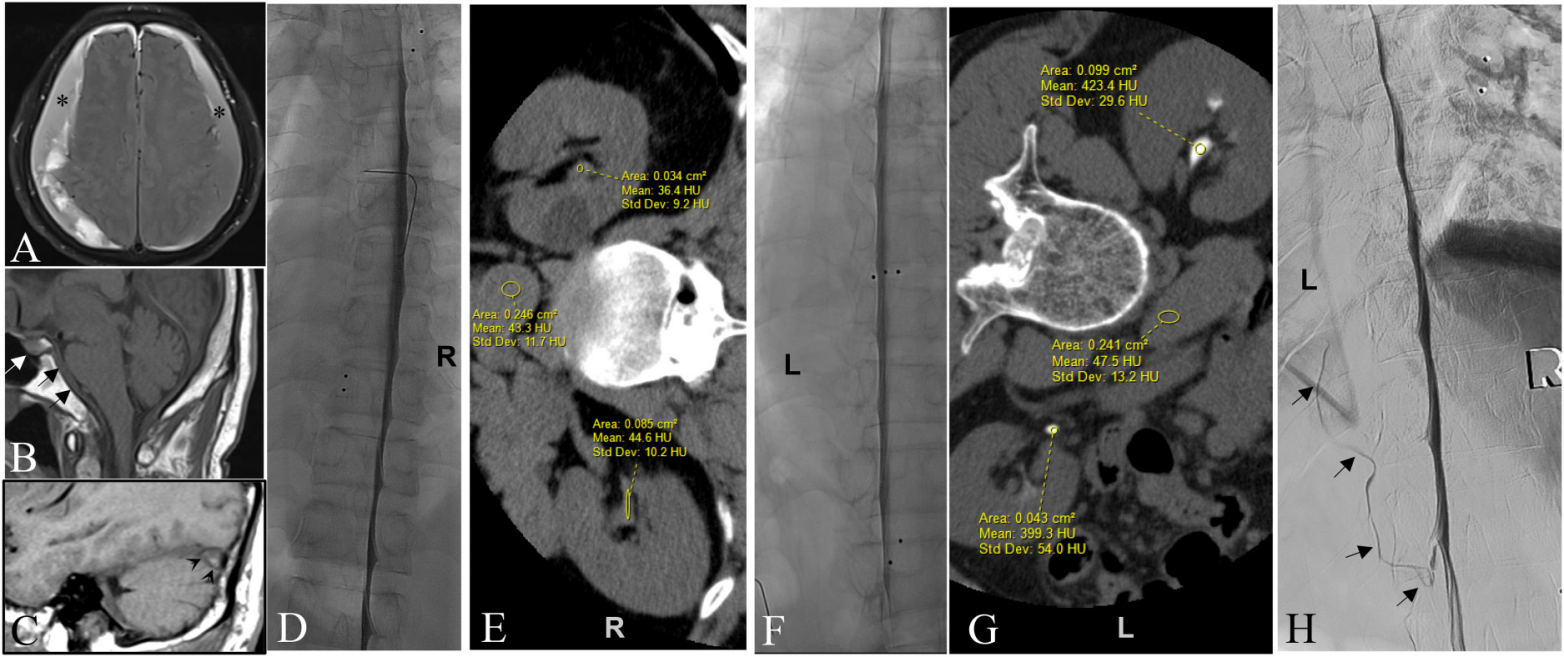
Patient 11. Showing differential rates of renal excretion associated with right versus left decubitus DSM–CT pair predicting sidedness of the culprit CVF . (A) Axial FLAIR image showing large bilateral subacute SDHs over the convexities of the brain (asterisks). (B and C) Midline and paramedian sagittal T1 images of the brain respectively showing enlargement of the pituitary (white arrow), dural thickening on the clivus (black arrows) and convex undersurface of the transverse sinus (venous distension sign) (arrowheads) as signs of intracranial hypotension despite the presence of bilateral subdural hematomas. (D) Right LDDSM showing no evidence of CVF. (E) Right decubitus CT performed immediately following the DSM showing only subtle contrast in the renal collecting systems measuring up to 45 HU . (F) Left LDDSM did not reveal a CVF. (G) Left decubitus CT performed immediately following the left LDDSM does show a high level of contrast agent within the renal collecting systems measuring up to 423 HU. The fact that contrast agent is seen in the renal pelvises immediately following DSM strongly suggest that a CVF is indeed present in this patient. Moreover, it is the disparity between these two decubitus measurements of renal contrast, i.e. much higher following the left LDDSM–LDCT pair, that further indicates that the CVF is most likely present on the left side even though it was not visualized on the original left LDDSM. This therefore compelled us to repeat left LDDSM. (H) Repeat Left LDDSM with the benefit of general anesthetic revealing the CVF arising beneath the left T10 pedicle ascending superiorly in a left paravertebral vein (black arrows).
